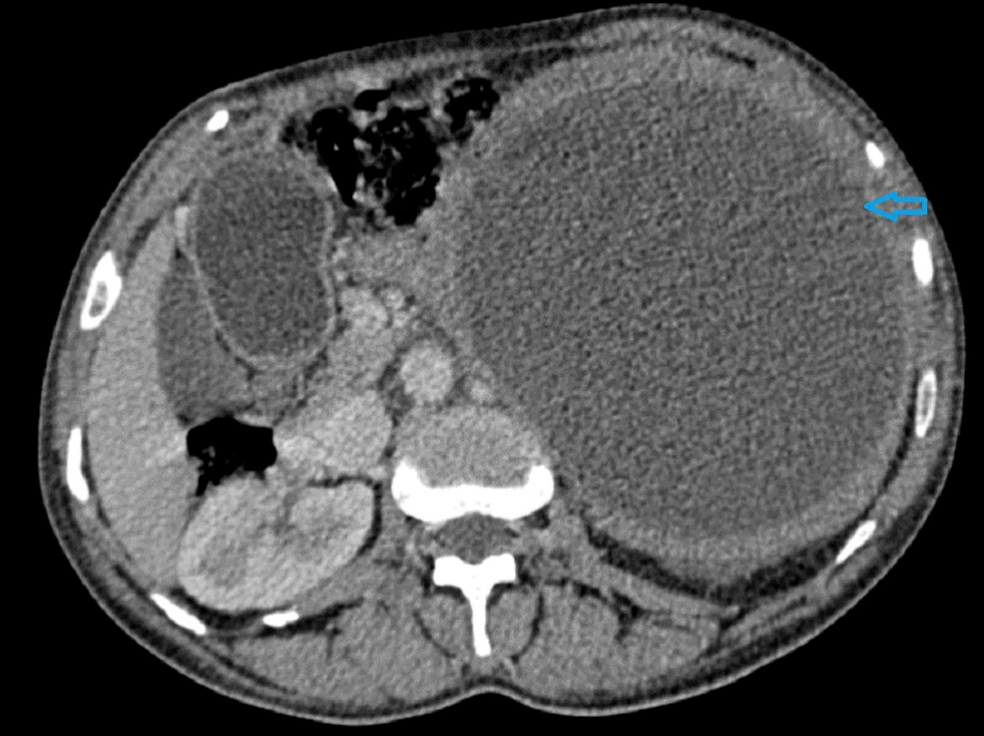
Abdominal computed tomography image The image is showing a well-defined, hypodense, retrogastric 20-cm mass with thickened wall and heterogenous enhancement.
